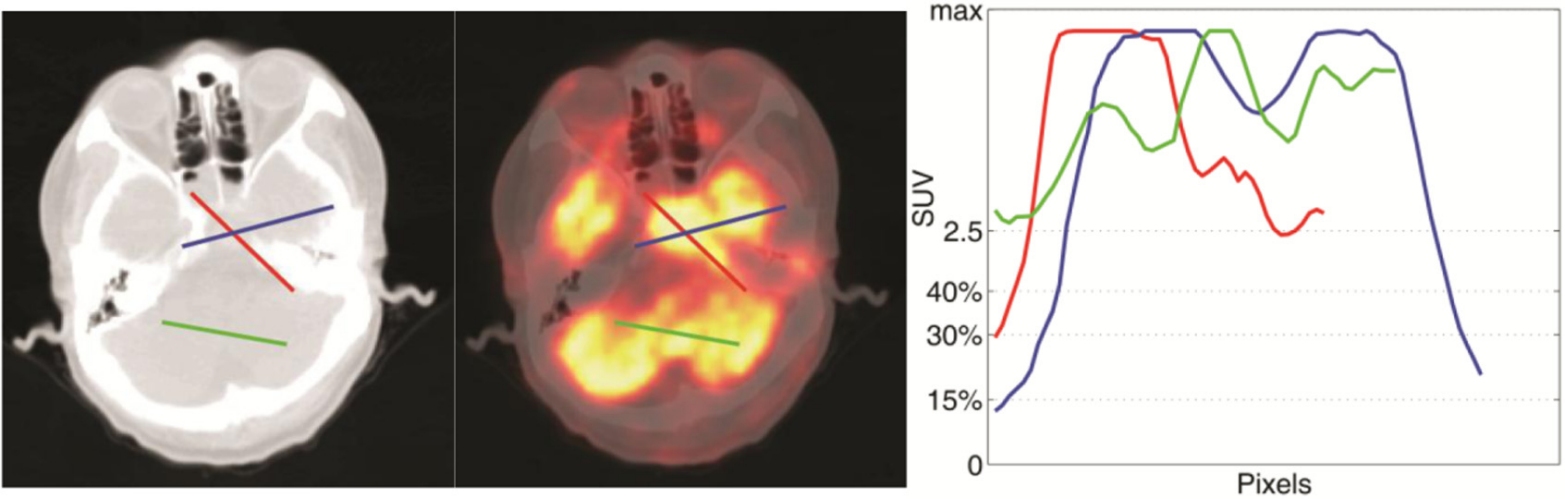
SUV of tumor and surrounding tissuse. On the left side of the figure, two lines are drawn along axial (blue) and radial (red) directions of the lesions. The SUV under the two lines are sampled and shown on the right. SUV_15%_, SUV_30%_, SUV_40%_, SUV_2.5_ baselines are shown along the Y axis. SUV of lesion and normal tissues reveals many overlapping regions.
